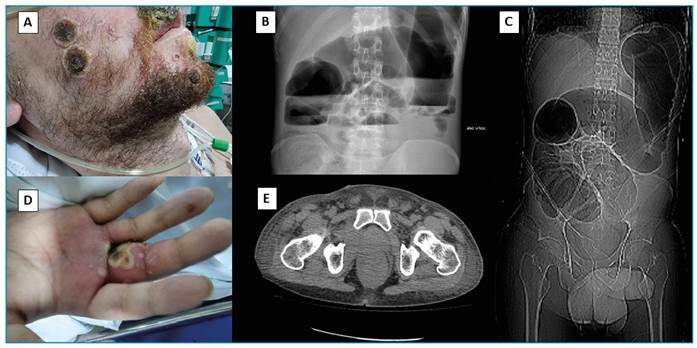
Ulcerated lesion with central necrosis and purulent border on the right cheek (A) and proximal phalanx on the upper lip of the third finger on the right hand (D). Abdominal radiography in orthostasis shows gaseous distension of the intestinal loops with Peer Review air-fluid level (B), and abdomen computed tomography shows distension of the colon, with heterogeneous content forming a level and irregular parietal thickening with reduced lumen transition between the sigmoid and the rectum (C and E).
